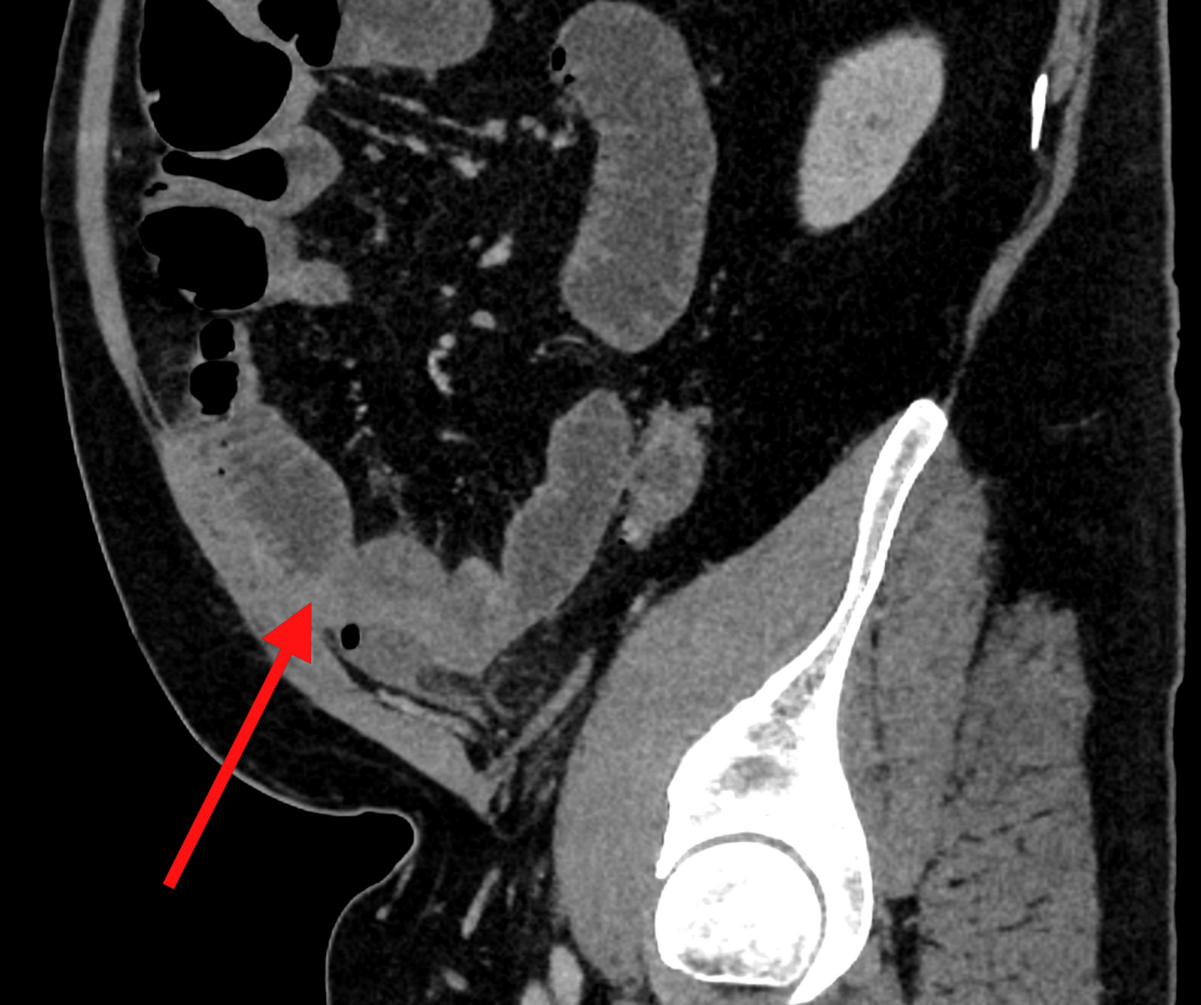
Sagittal view of contrast-enhanced CT of the abdomen The red arrow highlights the caliber change between dilated and collapsed small bowel loops, consistent with the transition point near the inflamed sigmoid colon.
